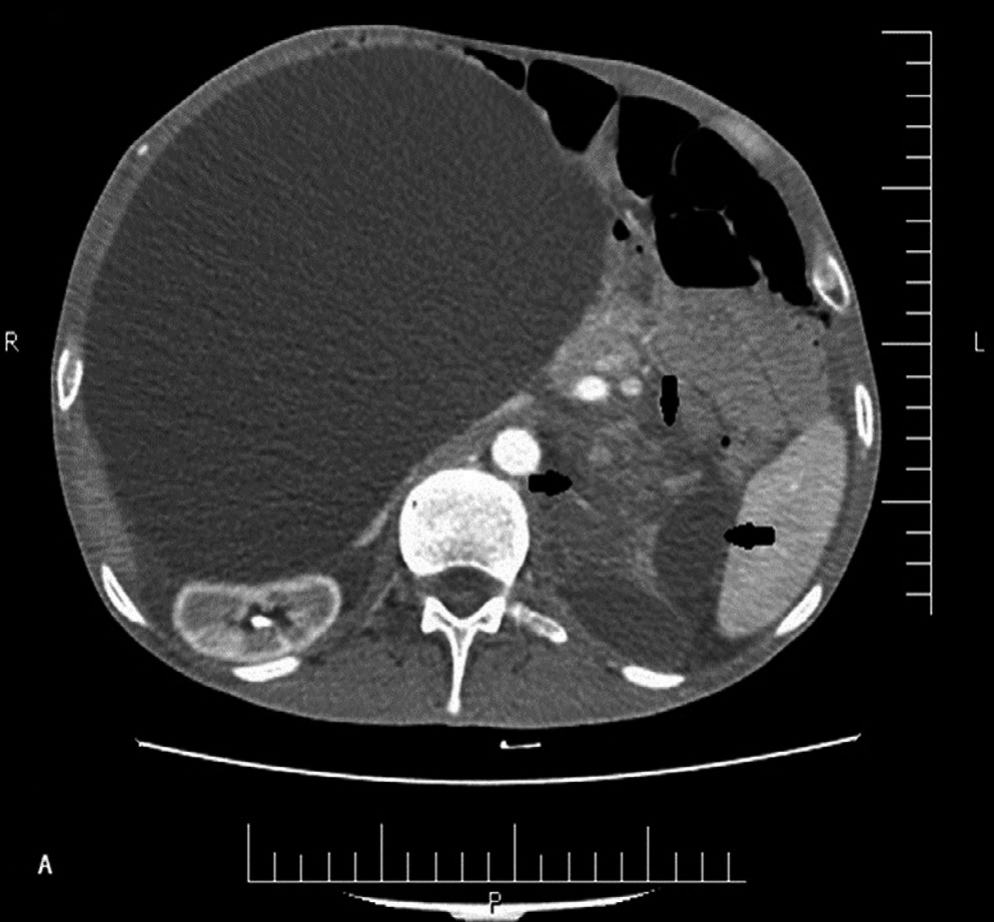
Abdominal computed tomography scan depicted retroperitoneal heterogeneous mass (black arrows)
